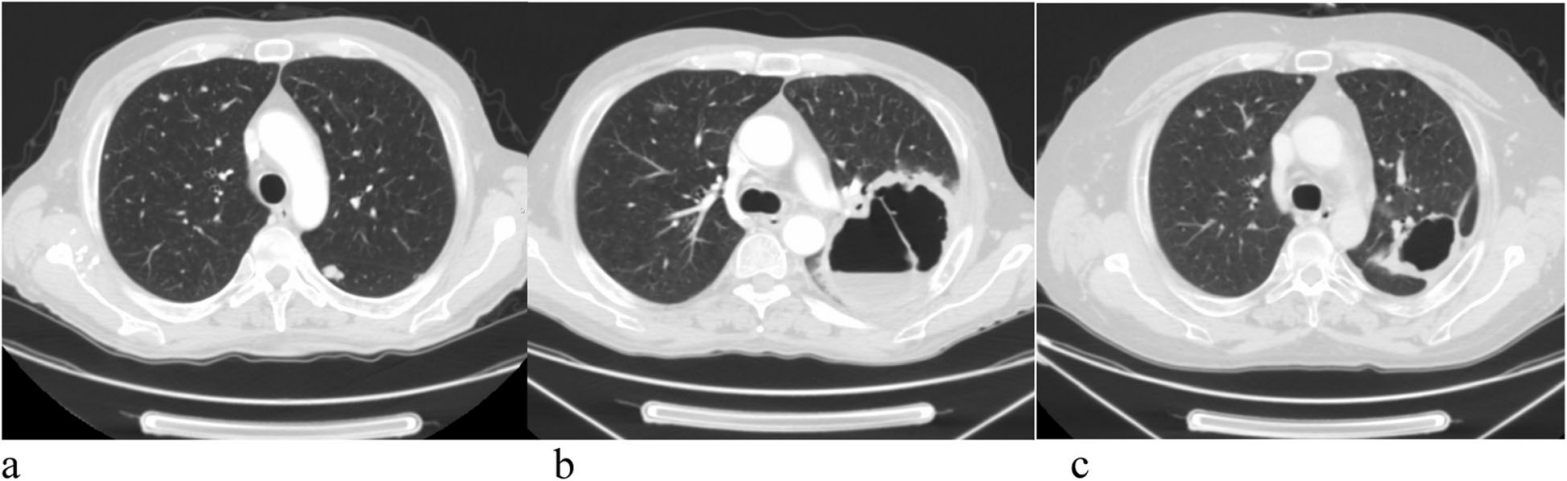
a Basal computed tomography. b Computed tomography slice showing necrotizing metastases with cavitation on lung similar to abscesses after 3 months of treatment. c Computed tomography slice showing resolution of abscesses 1 month after stopping treatment with pazopanib
